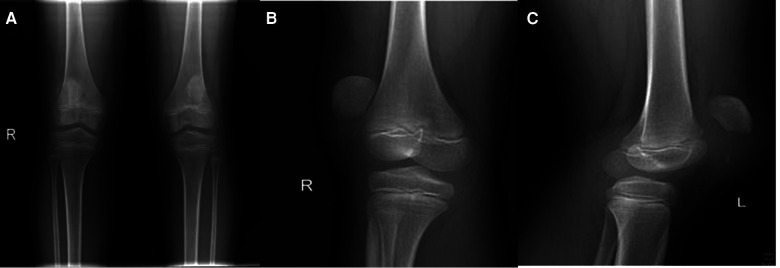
(A) AP radiograph of the both knees. (B) Lateral radiograph of the right knee. (C) Lateral radiograph of the left knee.
